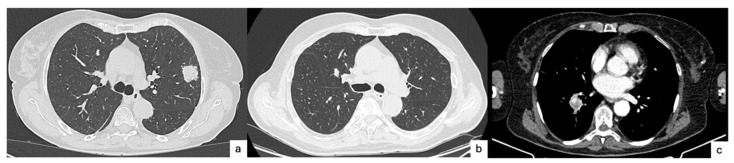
Three cases of STAS-positive lung cancers, as they appeared on CT scan. (a). Left upper lobe lung lesion presenting as solid lobulated nodule undergoing lobectomy, later revealed to be invasive lung adenocarcinoma pT2a pN0, STAS-positive. (b). Right upper lobe lung lesion presenting as solid lobulated nodule undergoing lobectomy, later revealed to be invasive lung squamous cell carcinoma pT1b pN0, STAS-positive. (c). Right lower lobe lung lesion presenting as solid nodule with central low attenuation (mediastinal window) undergoing lobectomy, later revealed to be invasive lung adenocarcinoma pT2a pN0, STAS-positive.
